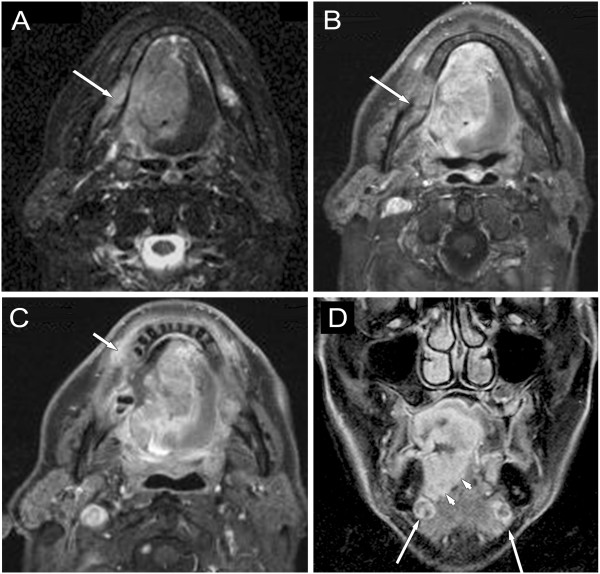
Oral cavity cancer, located in the sublingual region, stage T4aN2c. A: axial STIR-, B, C: axial post-contrast T1-weighted MRI images with fat suppression, D: coronal contrast-enhanced T1-weighted MRI images with fat suppression: demonstrate expansive carcinoma of the tongue infiltrating the gingiva (C image, short arrow), crossing the midline, invading the right mandibular base (A, B images, long arrows) and involving the extrinsic muscles of the tongue (D image, arrow-heads). On the coronal image bilateral small, well-defined submandibular lymph nodes are seen with sign of colliquation.
